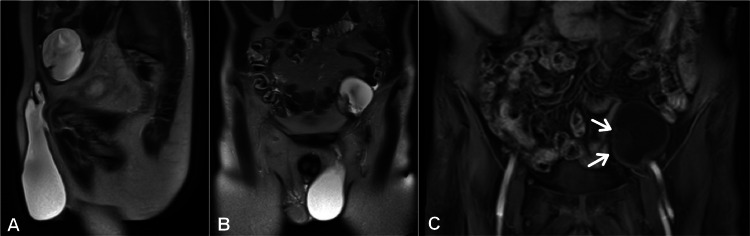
Abdominal and pelvic MRI image showing an ASH and UDT. (A) Sagittal and (B) Coronal T2-weighted MRI images showing dumbbell-shaped appearance of ASH. (C) Coronal contrast-enhanced T1-weighted MRI image showing an intracystic, atrophic and hypovascular testis (white arrows).
